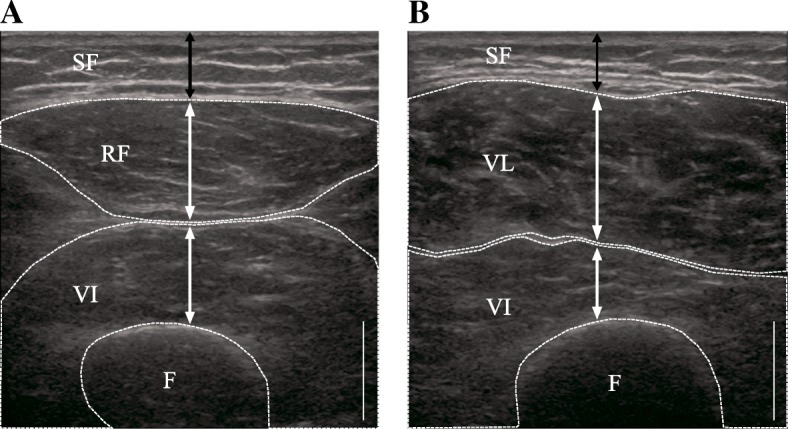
Representative ultrasound images of the anterior (a) and lateral (b) thighs. SF, subcutaneous fat; RF, rectus femoris; VI, vastus intermedius; VL, vastus lateralis; F, femur. Black double-headed arrows show subcutaneous fat thickness. White double-headed arrows show muscle thickness. Scale is 1 cm
